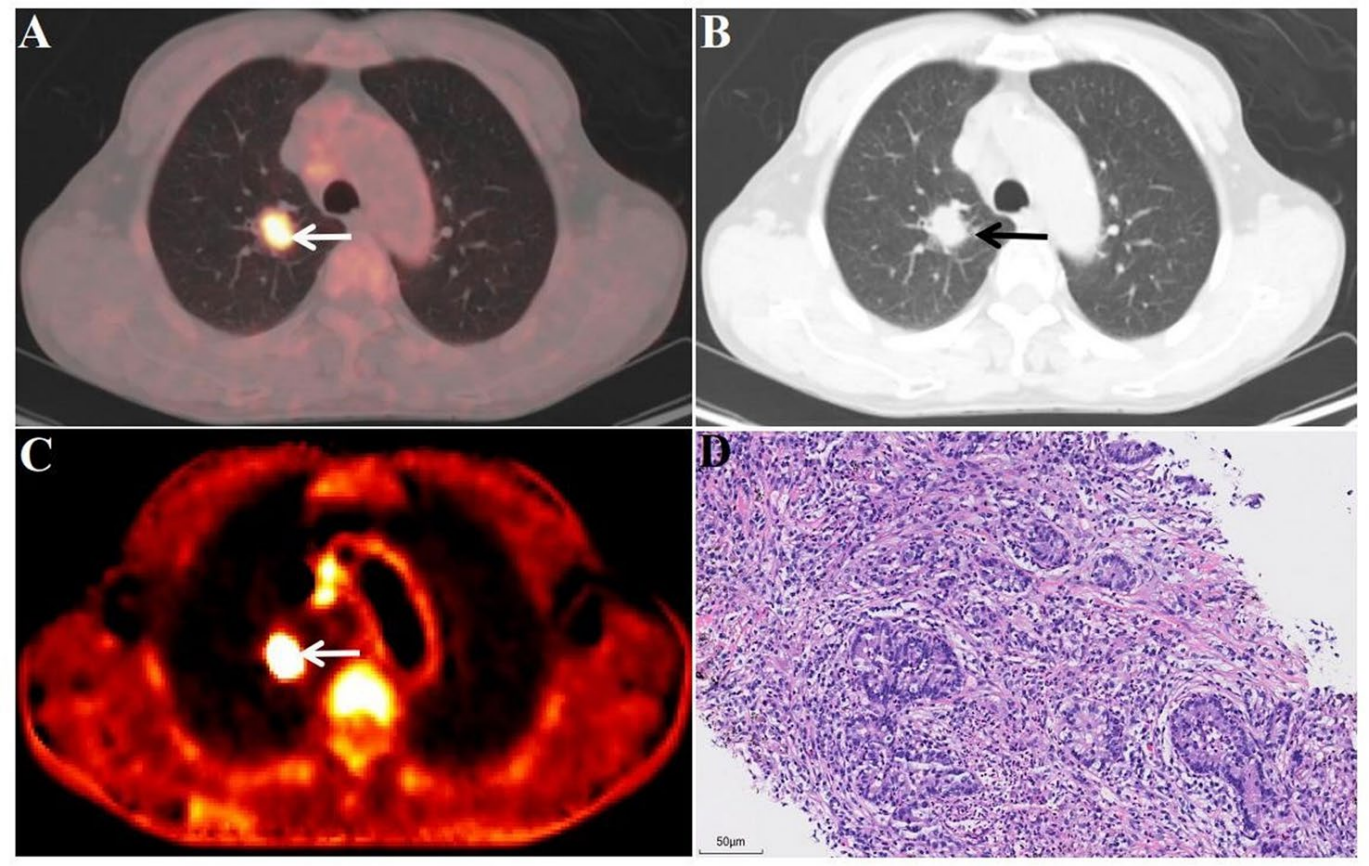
FDG-PET/CT images of a malignant lesion A 59-year-old male patient. Surgical pathology confirmed an adenocarcinoma (D, 20x field of view) in the lower lobe of the right lung (white/black arrow), with a size of 2.0 × 1.6 cm (A and B, white/black arrow), SUV_max_ of 7.9 (A, white arrow), and K_i_ of 0.0442 ml/g/min (C, white arrow). Postoperative EGFR testing was negative
